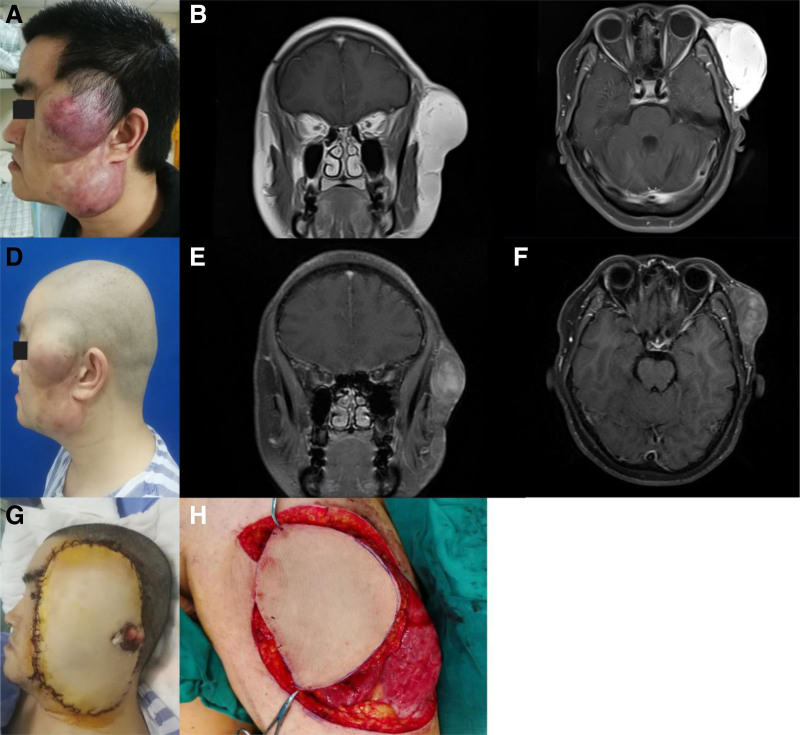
Clinical presentation and MRI findings of case 1. (A) DFSP lesion located in the face at baseline; (B–C) Magnetic resonance imaging prior to the beginning treatment with imatinib mesylate; (D) DFSP lesion after treatment with imatinib mesylate; (E–F) Magnetic resonance imaging after treatment with imatinib mesylate; (G) Surgical region after resection and reconstruction using free latissimus dorsi flap; (H) Harvested free anterolateral thigh flap.
